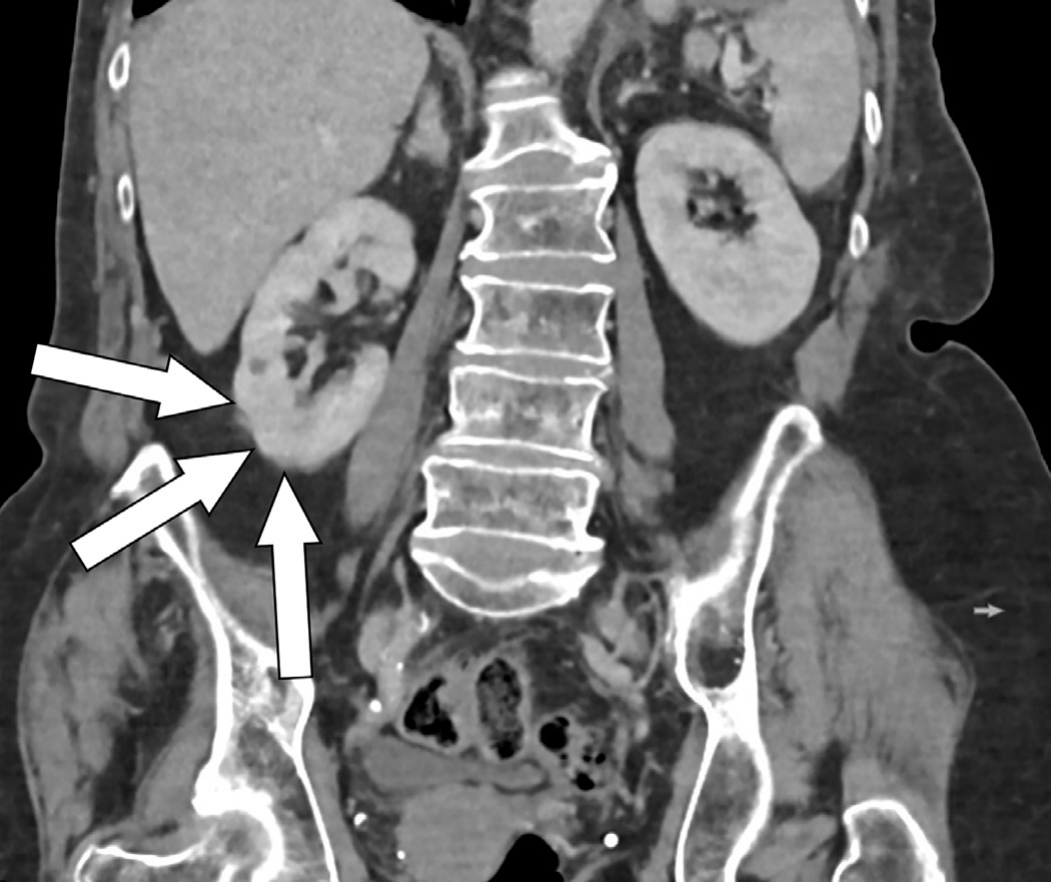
Follow-up CT on April 9, 2024 showing no sign of evidence of a tumor in the right kidney (white arrows). Contrast-enhanced abdominal CT in venous phase, coronal reformation.
